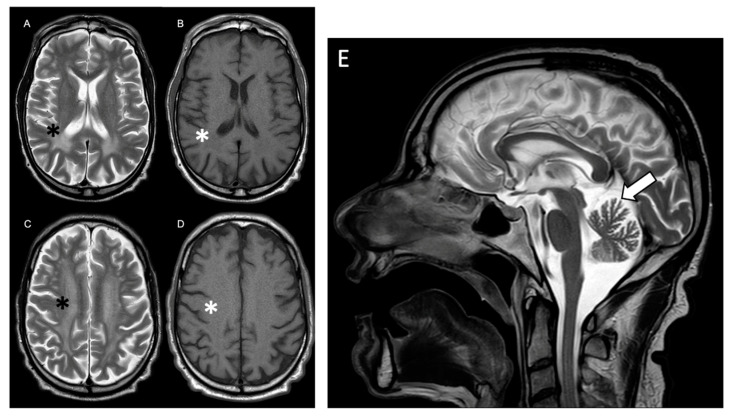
Panels showing T2-weighted (A,C) and T1-weighted (B,D) axial brain MRI scans at the basal ganglia (A,B) and corona radiata (C,D) level. An extensive and symmetric T2 hyperintensity (A,C) black asterisks involving mainly occipital and parietal white matter was observed, with corresponding T1 slightly hypointense signal (B,D) white asterisks, sparing subcortical U-fibers and corpus callosum. T2-weighted sagittal brain MRI scans (E) showing mild vermian cerebellar atrophy (white arrow).
